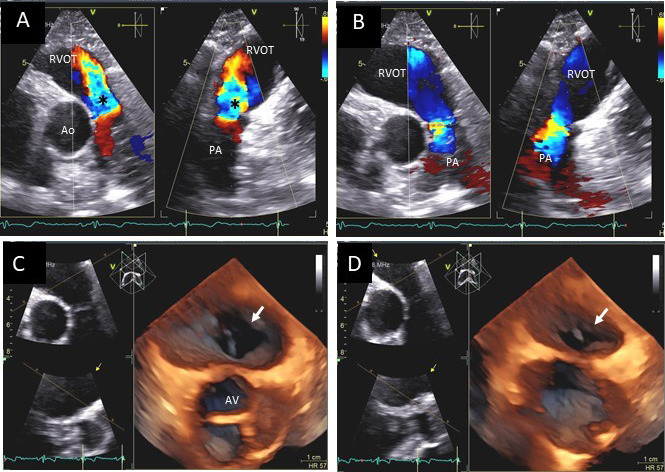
Pulmonic valve regurgitation and stenosis. Transthoracic
